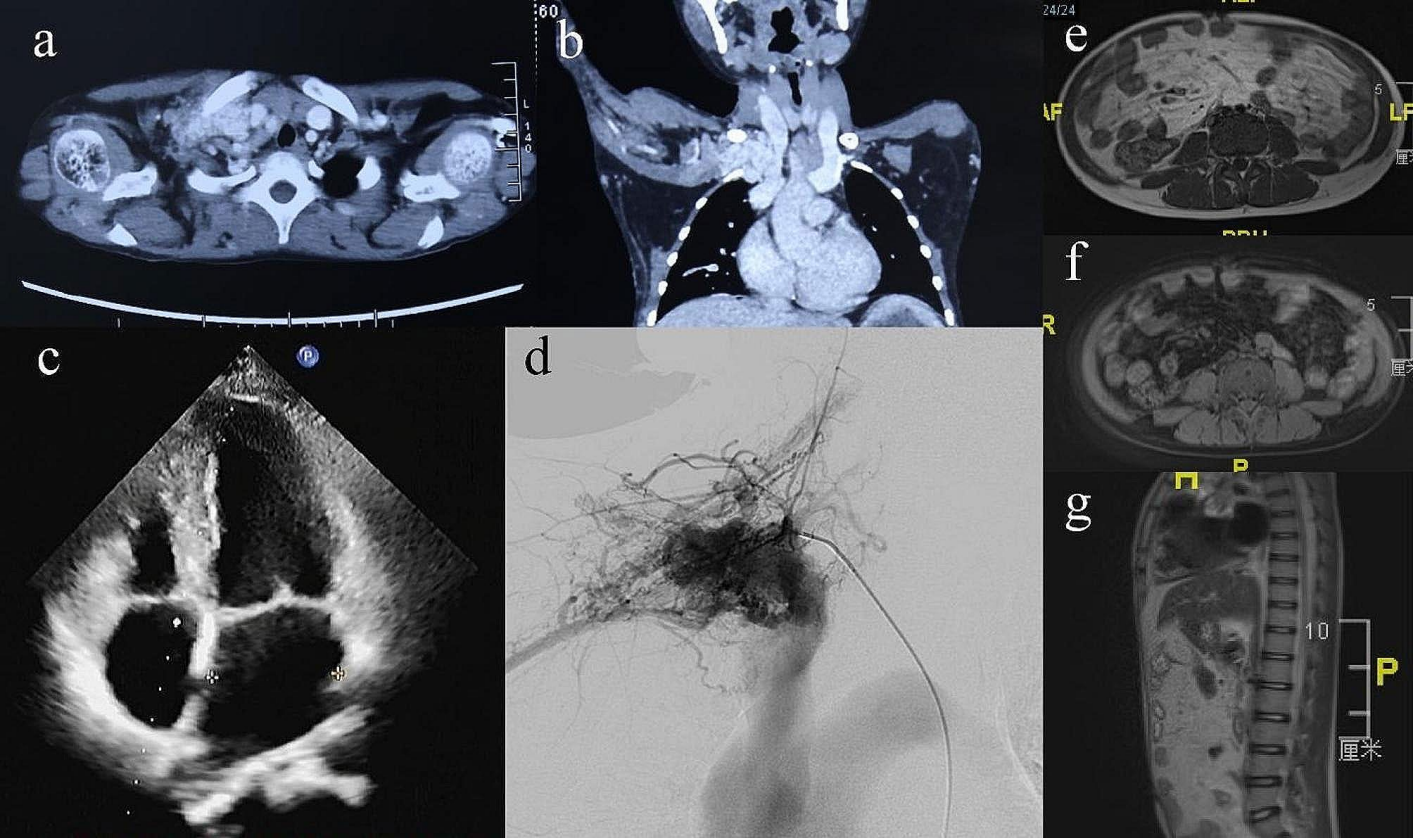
Computed tomography (CT), echocardiography, digital substraction angiography (DSA) and magnetic resonance imaging (MRI) images of the patient. (a-b) CT scan (coronal and axial images) showed a large AVM on the right cranial thoracic entrance and enlargement of the cardiac shadow. (c) echocardiography demonstrated an enlarged left ventricle and left atrium. (d) DSA showed a high-flow AVM, fed by the right subclavian artery and its branches. (e-g) MRI (T1WI axial, T1WI axial with fat-suppression and T1WI sagittal images) showed diffuse fatty infiltration of the abdomen
